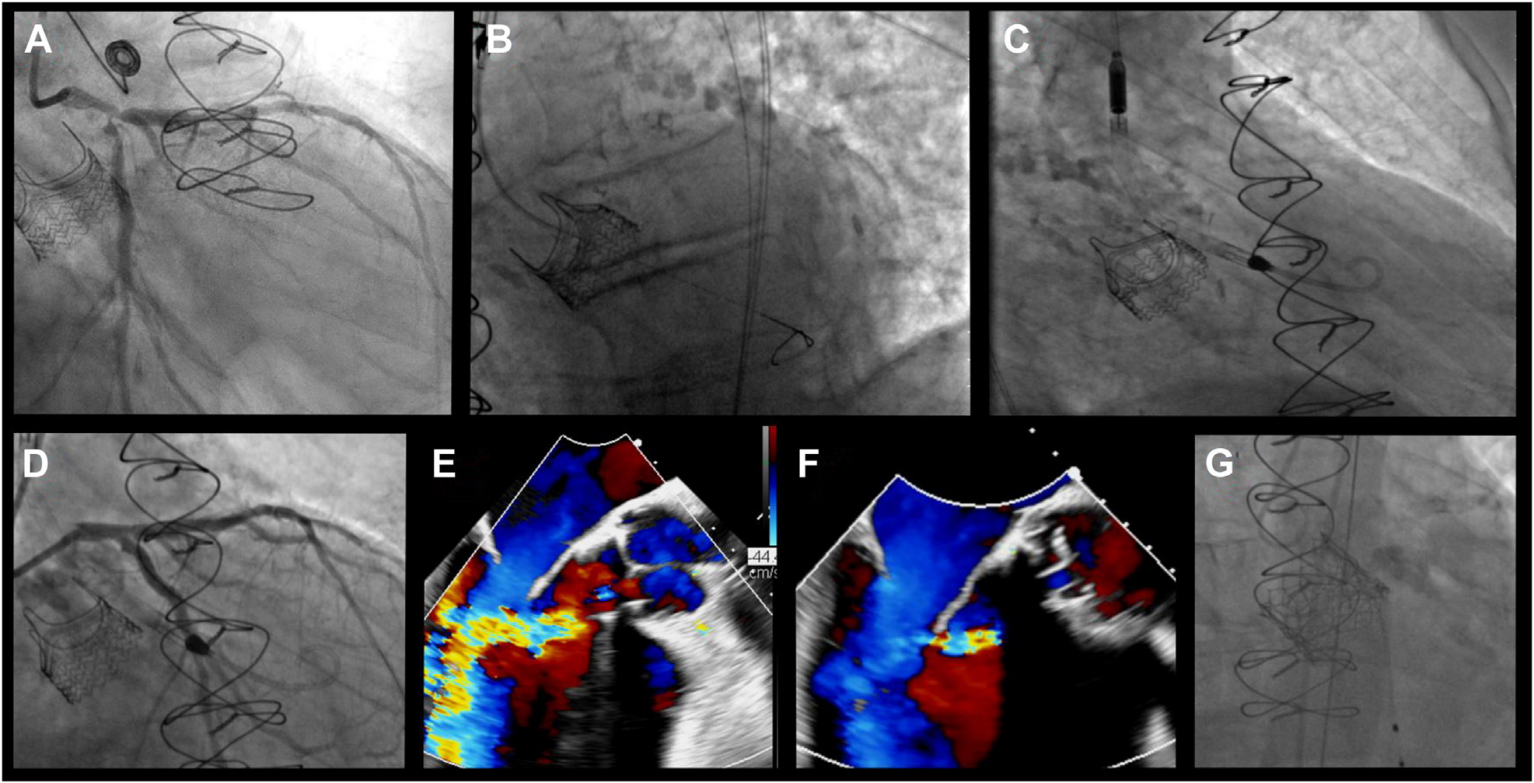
Angiography and echocardiography imaging of the case. (A) Diagnostic coronary angiogram. (B) Left anterior oblique view of the wire into the left ventricle. (C) Right anterior oblique view after the Impella was placed into the left ventricle via the paravalvular channel. (D) Final angiogram after percutaneous coronary intervention. (E) Transesophageal echocardiogram showing severe aortic insufficiency after removal of Impella. (F) Transesophageal echocardiogram after valve-in-valve implant. (G) Aortic root angiography showing minimal aortic insufficiency.
